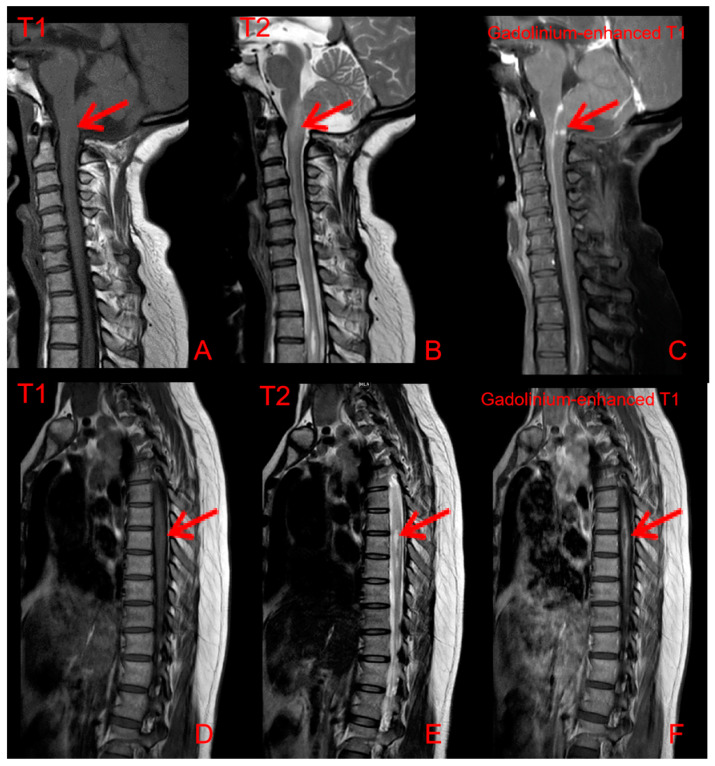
MRI of the spinal cord shows (A) hypointensity T1 signal and (B) hyperintensity T2 strips lesions below the level of the medulla oblongata, with slight thickening and swelling of the spinal cord. (C) Gadolinium-enhanced T1 MRI shows the line-like and nodular enhancement lesions at the level of C1 (SE 601A; IM 6), about 3 mm. (D) T1 phase, (E) T2 phase, and (F) gadolinium-enhanced T1 MRI shows the syringomyelia at the T3-4 level.
